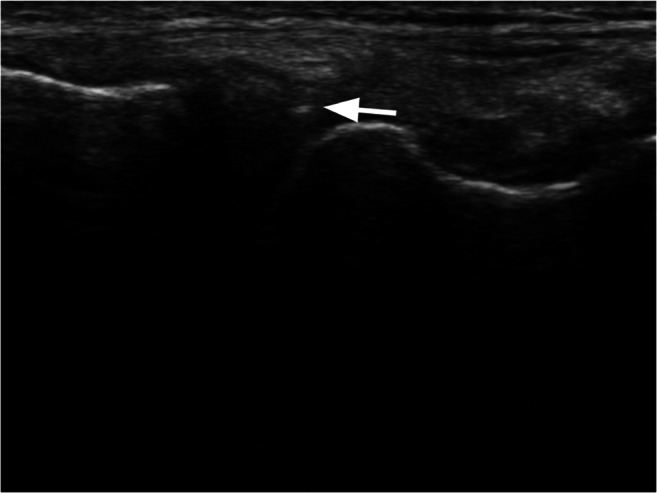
Needle tip visualized in an out-of-plane view for MFAT injection into the meniscus under ultrasound guidance
